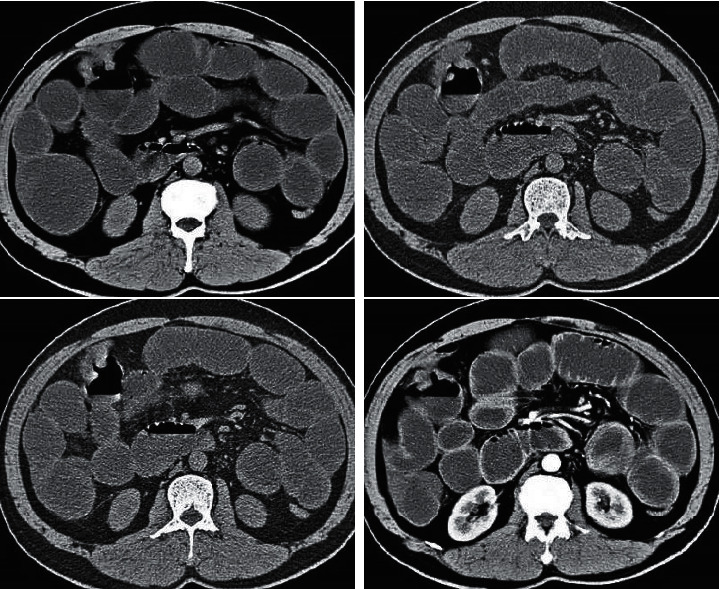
CT image of a female patient at the age of 55. She complained mainly about stomachache and abdominal distention, which lasted for over one month. Besides, there was no obvious incentive more than one month before admission to hospital. However, she suffered from discontinuous abdominal distention and peripheral umbilicus paroxysmal colic along with nausea and emesis. Vomitus was excreted from stomach. After emesis, abdominal pain was alleviated to some extent along with belching and heartburn.
